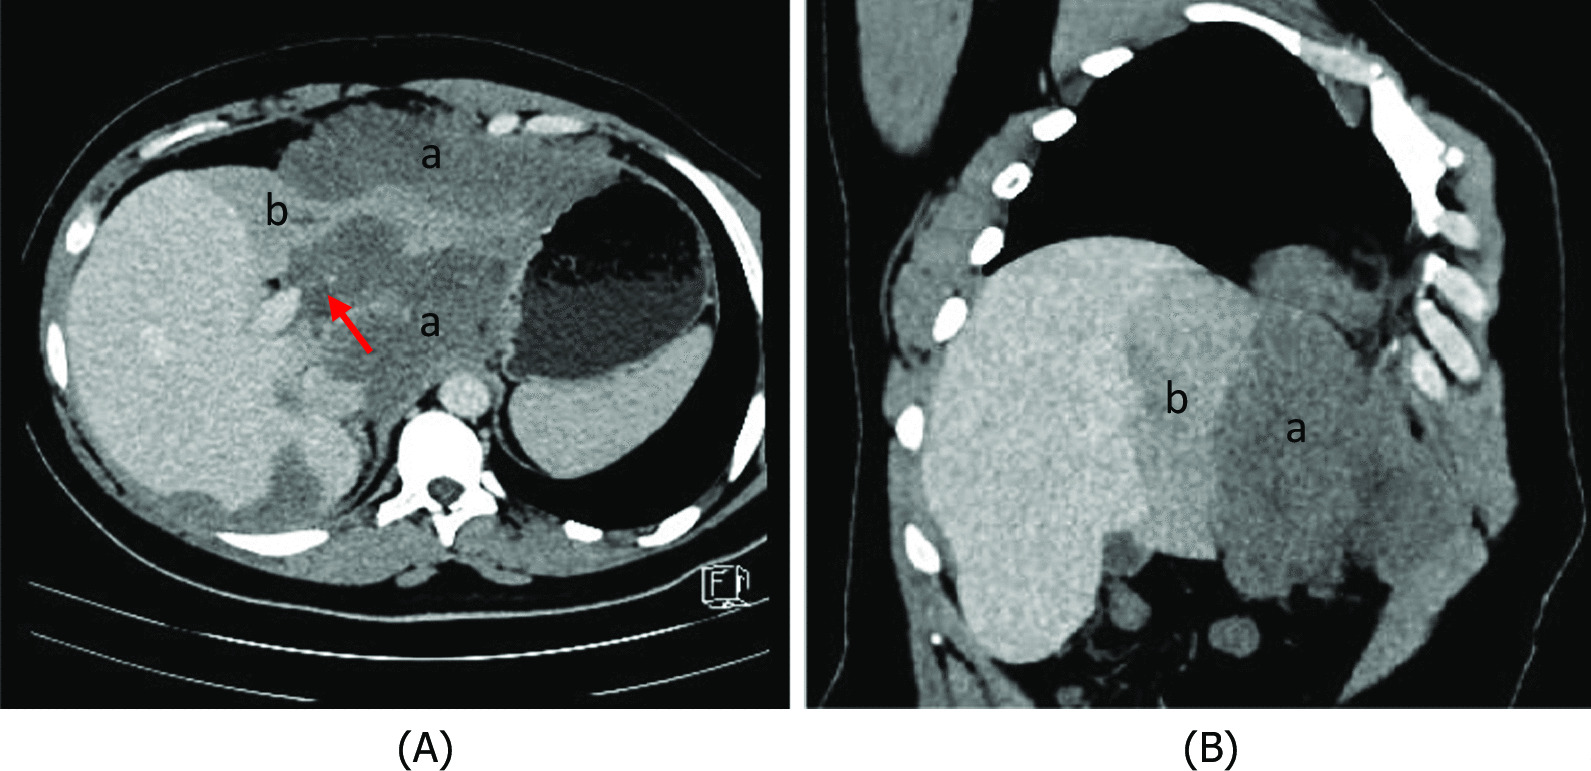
Case 3: axial (A) and coronal (B) sections of abdominal CT scan with injection at venous time, showing tissue mass (a) responsible for scalloping on the left liver and hepatic hilum with obstruction of the left portal branch (red arrow) causing hypoperfusion of the left liver (b)
